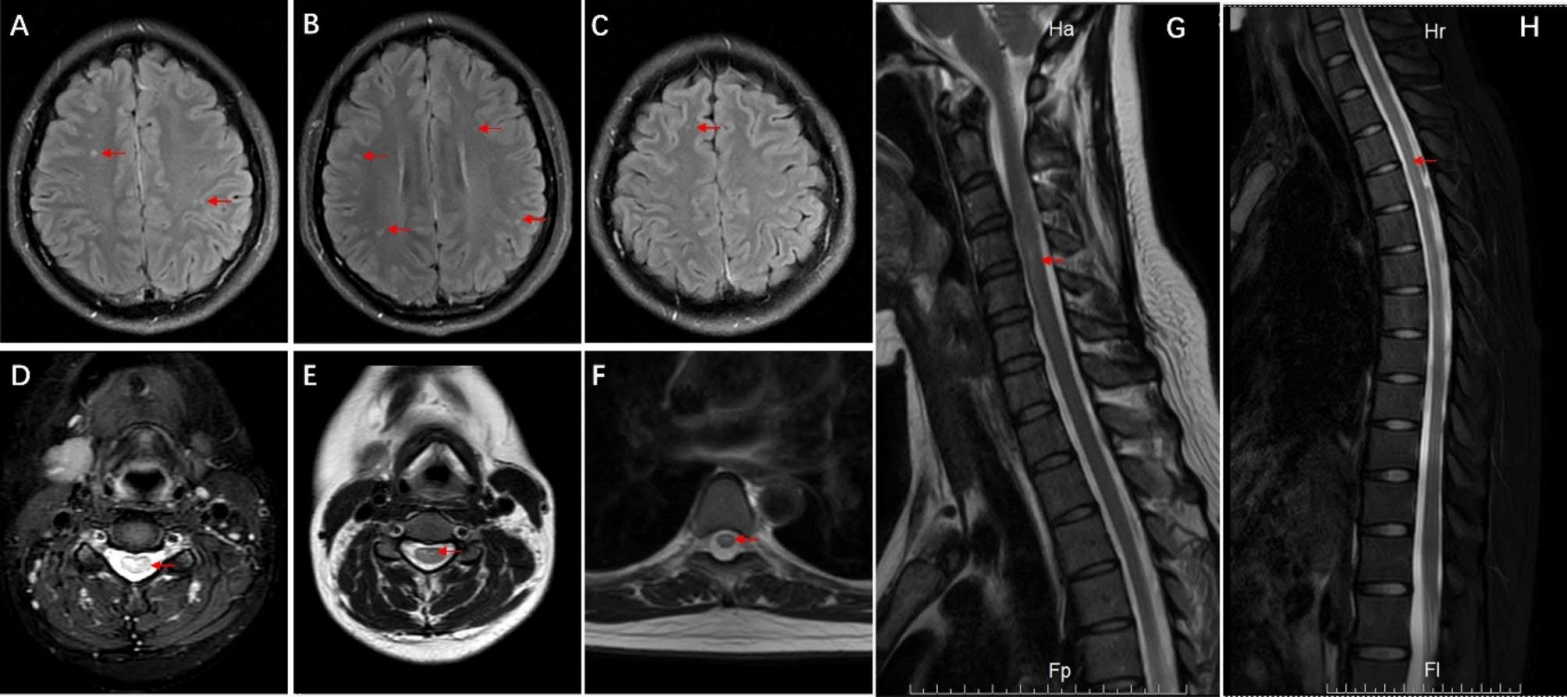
Brain MRI (T2-FLAIR) performed in November 2021 shows multiple hyperintense white matter lesions adjacent to the bilateral ventricle, at the bilateral frontal lobe, and at the right centrum semiovale and venous developmental anomaly at the left frontal lobe (A–C). DWI shows no abnormalities. Axial cervical (T2) and thoracic (T2-FS) spinal MRI present multiple hyperintense patchy lesions along the cervical and thoracic segments (D–F). Sagittal MRI (T2–FS) shows banded lesions along the thoracic and cervical cord at the C4–C6 and T2–T9 levels (G, H). Strong signals are not present on enhancing MRI. Red arrowheads indicate the lesions. DWI, diffusion-weighted imaging; MRI, magnetic resonance imaging; T2, T2-weighted imaging; T2-FLAIR, T2-weighted fluid-attenuated inversion recovery imaging; T2-FS, fat-saturated T2-weighted imaging
